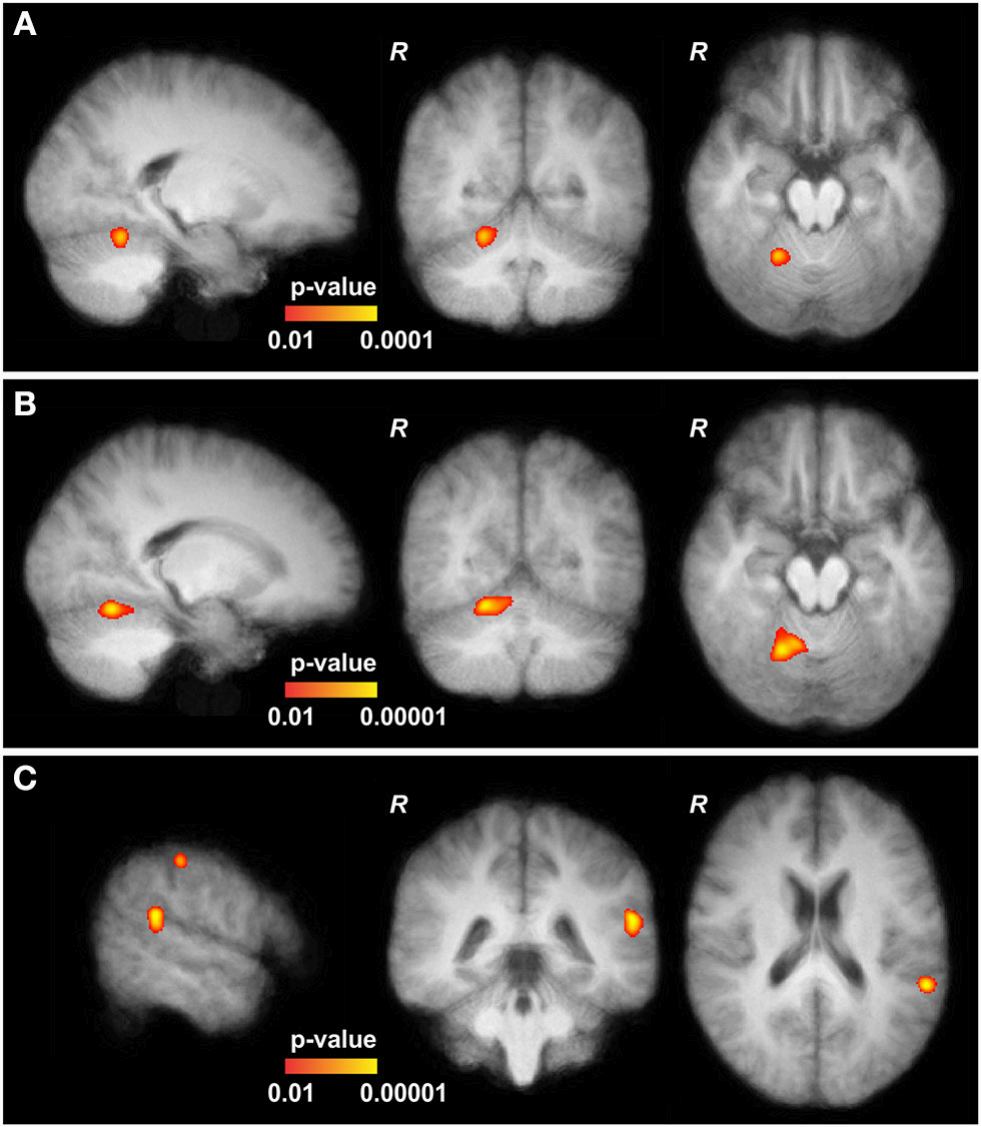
Functional MRI data of cervical dystonia (CD) patients. (A) At baseline, the upper part of the cerebellum (CRB) of CD patients showed slightly increased activation in comparison to controls (MNI152 21, −54, −18). (B) After continuous theta-burst stimulation (cTBS), main and significantly increased activations in CD patients are shown in the upper part of the right CRB (MNI152 19, −59, −16, adjacent to the baseline results, further pronounced). (C) Two other significantly elevated activations were found adjacent to the gyrus angularis (MNI152 −57, −42, 21) and the postcentral sulcus (MNI152 −55, −27, 48). All depicted activations are overlayed on the average coregistered and linearly transformed brains of the subjects. Some moderate but significantly elevated activations in the left primary motor and primary somatosensory cortex and the left premotor cortex are not shown. Comparison with patients at baseline and after stimulation of the left dorsal premotor cortex (CRB vs. PMd) showed increased activations at the same locations and at the same significance levels with only minimal differences (not shown).
